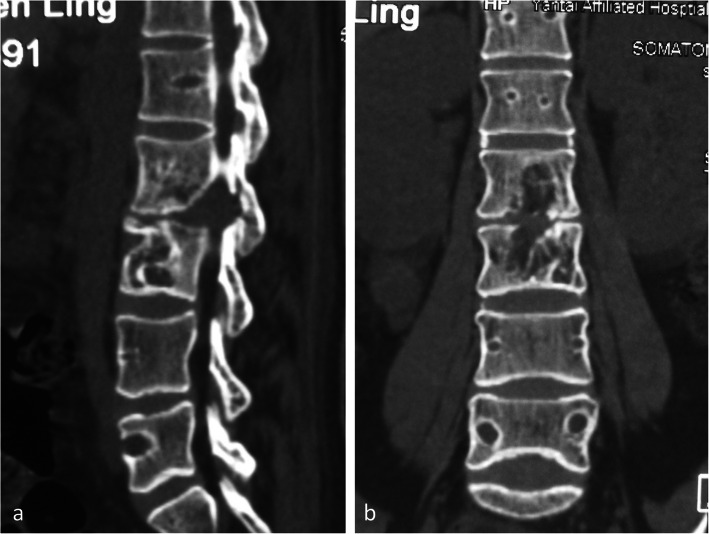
A 28-year-old girl with drug-resistant tuberculosis, sagittal (a) and coronal (b) CT reconstruction after instrumentation removal at the third year follow-up, showed no signs of intervertebral fusion, but the periphery healing of the vertebrae and preservation of bilateral facet joints provide sufficient support to anterior and posterior column to ensure the spinal stability
